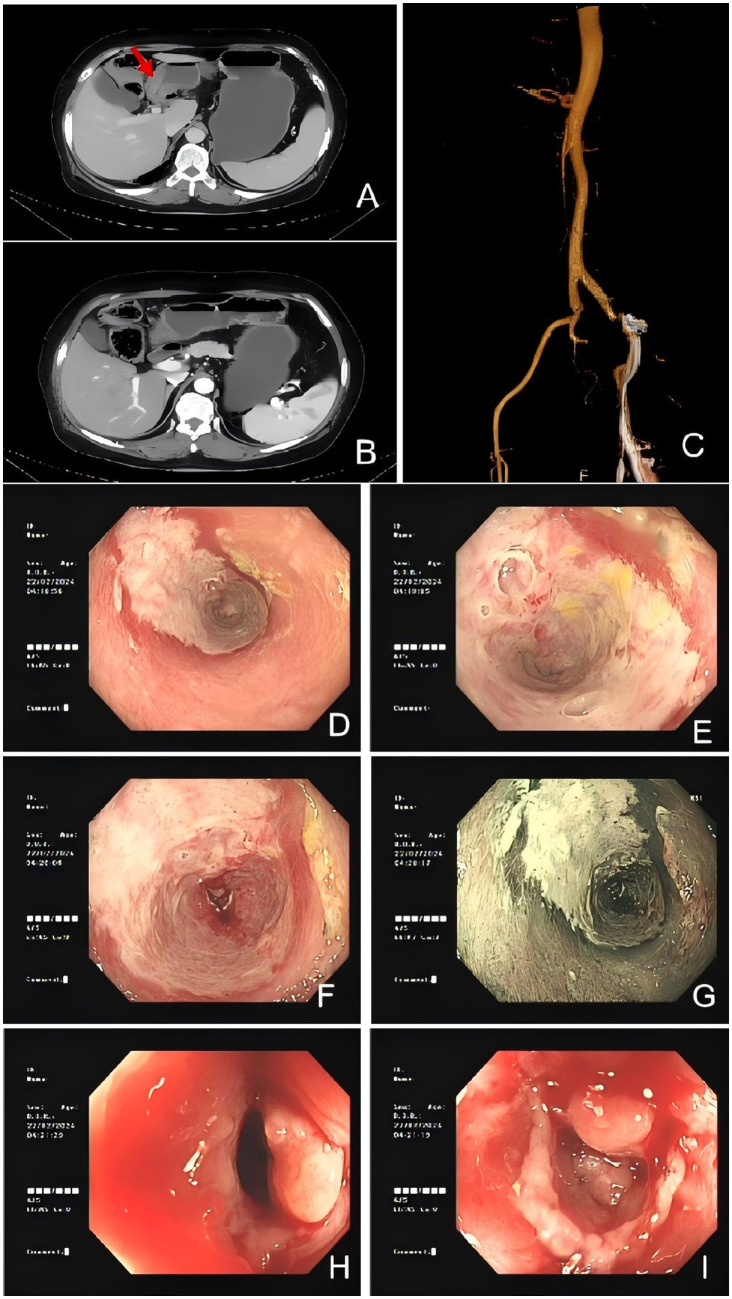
Colonic imaging and computed tomography suggested IMHMV. (A,B) Axial CT images showing thickened colonic wall with edema (red arrowhead) and no mesenteric artery stenosis. (C) Abdominal CTA revealed mixed plaques at the origin of the abdominal aorta and bilateral common iliac arteries, suggesting the possibility of mesenteric microthrombosis. Flexible sigmoidoscopy images demonstrated severely inflamed mucosa (D–J) with significant luminal narrowing (H,I).
